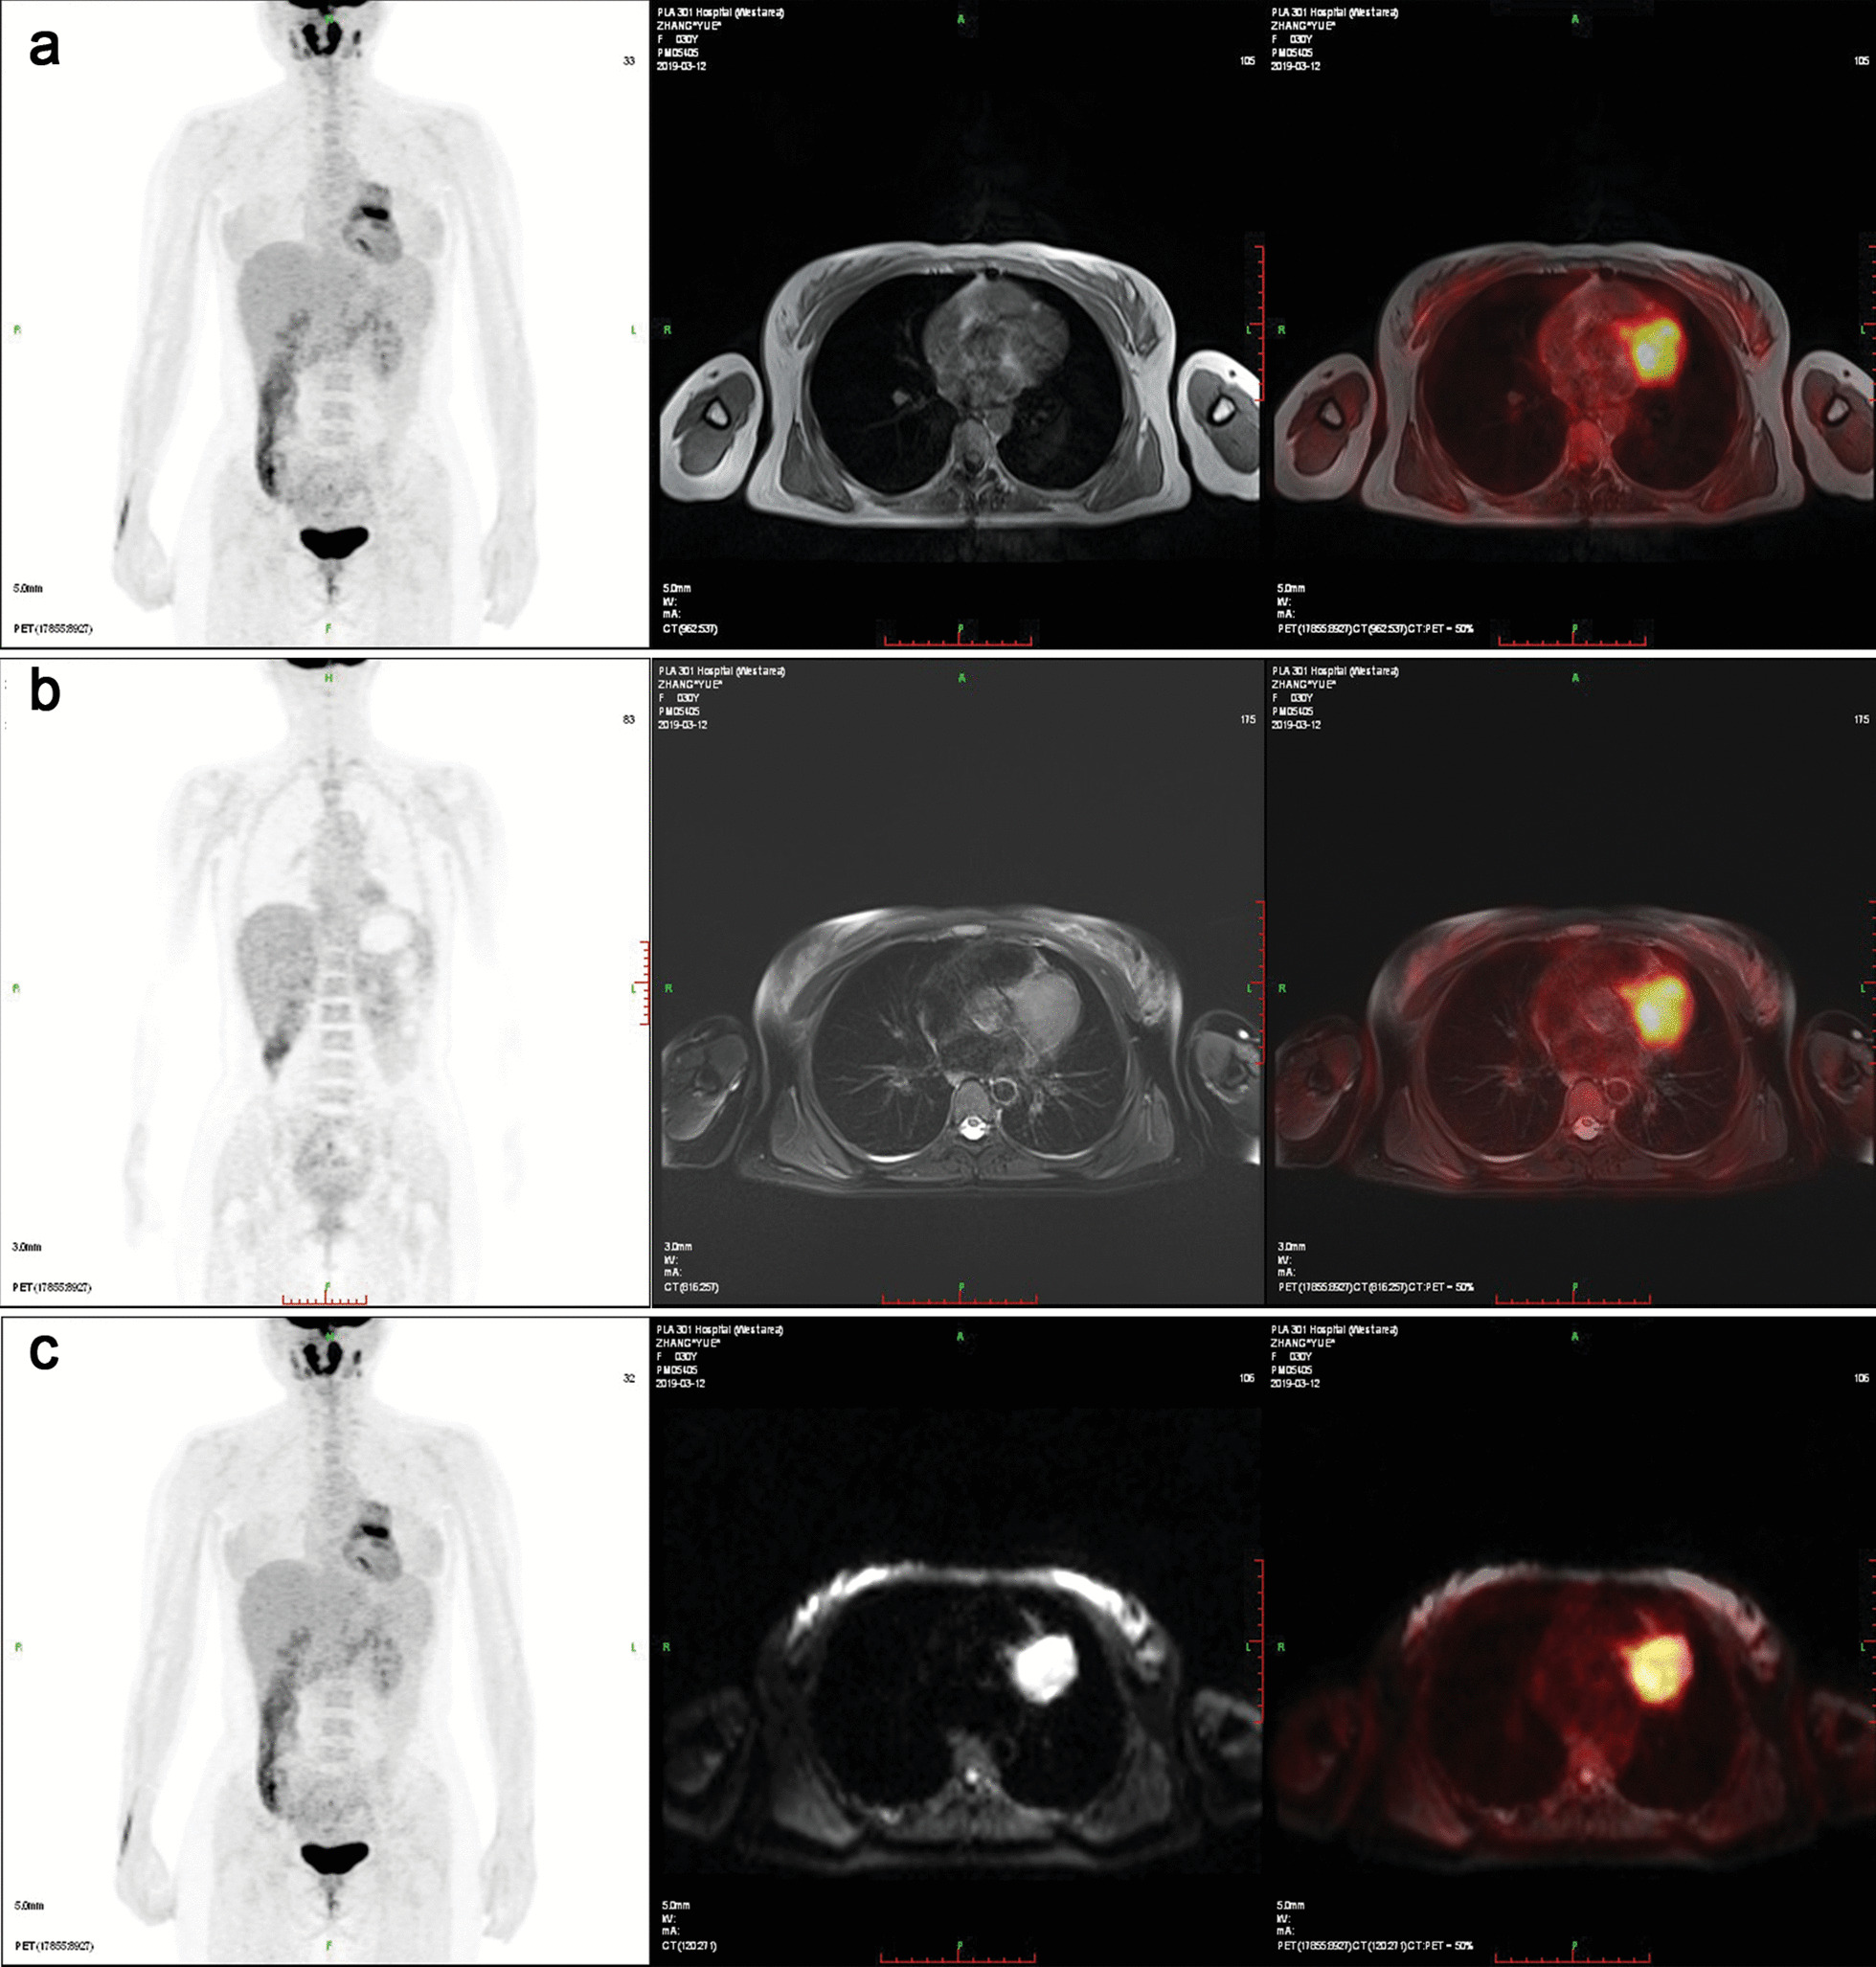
The imaging characteristics of PNET on PET/MRI at recurence. Positron emission tomography-MRI shows an irregular soft-tissue mass in the pericardial cavity with apparent higher metabolism. a The lesions showed iso-intense signals on T1WI. b The lesions showed hyperintense signals on T2WI. c The lesions showed significantly inhomogeneous hyperintense signals on the DWI
